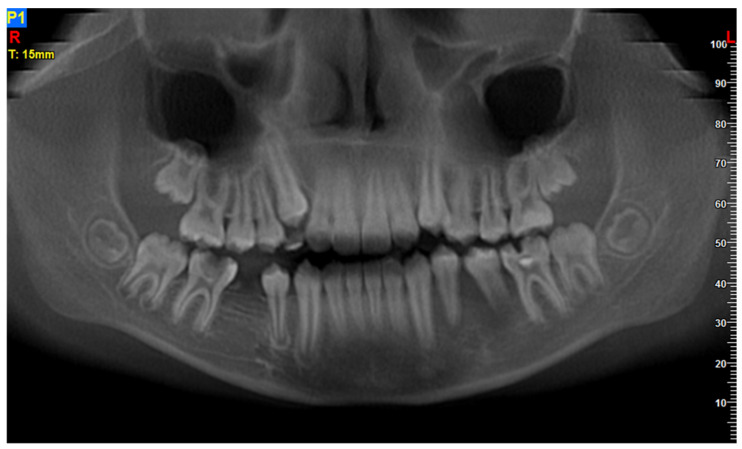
Patient, 11 y.o.: Panoramic reconstruction from CBCT examination. Rarefication of cancellous bone in the apical region of tooth 35 with apical resorption and the appearance of “floating in the air”.
